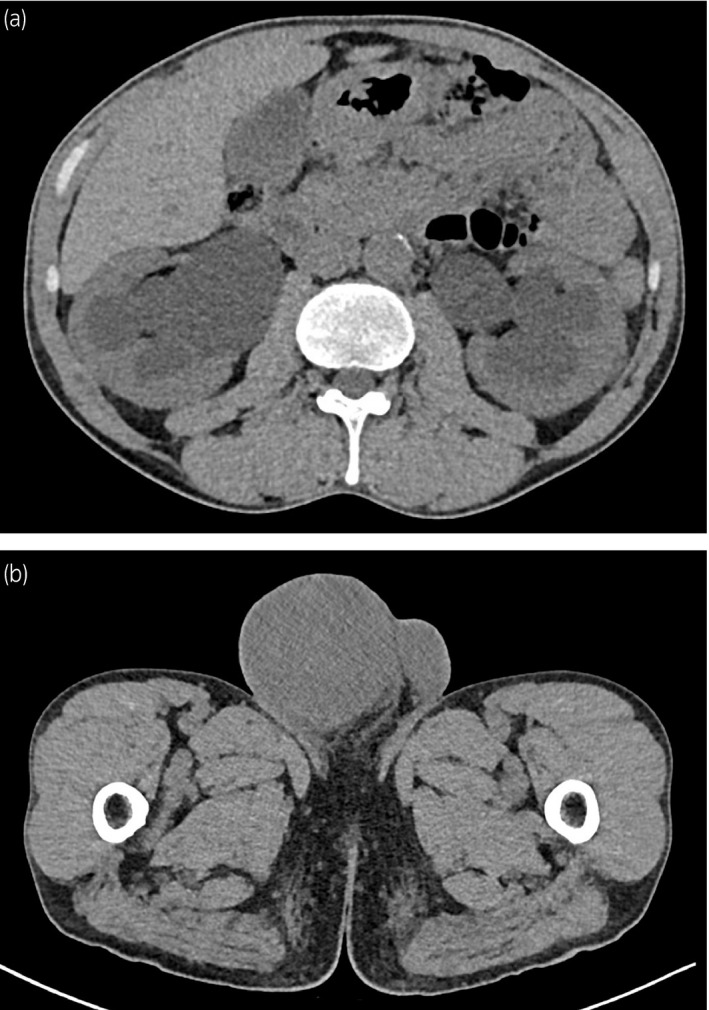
(a) CT scan showing bilateral hydronephrosis worse than a year ago. (b) CT scan showing an about 10 cm right intrascrotal mass.
